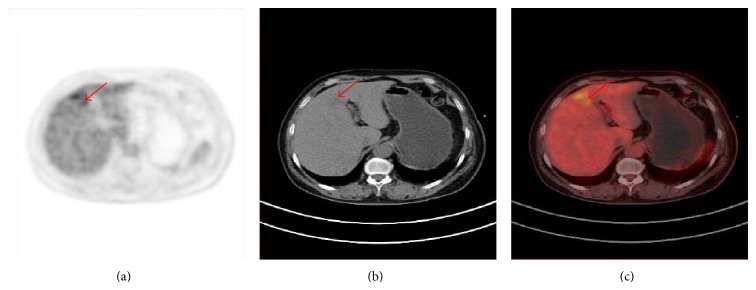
PET, CT and PET/CT of a 59 year old patient, referred for restaging because of elevated CA 15-3 level 12 years after surgically resected breast carcinoma of the left breast. The PET demonstrates a focal increased FDG uptake in the liver (red arrow), which is considered as malignant. The CT shows a slightly low dense area in the left lobe of liver. The PET/CT is considered as suspicious because of the PET findings. Metastatic involvement of the liver was proved by follow-up.
